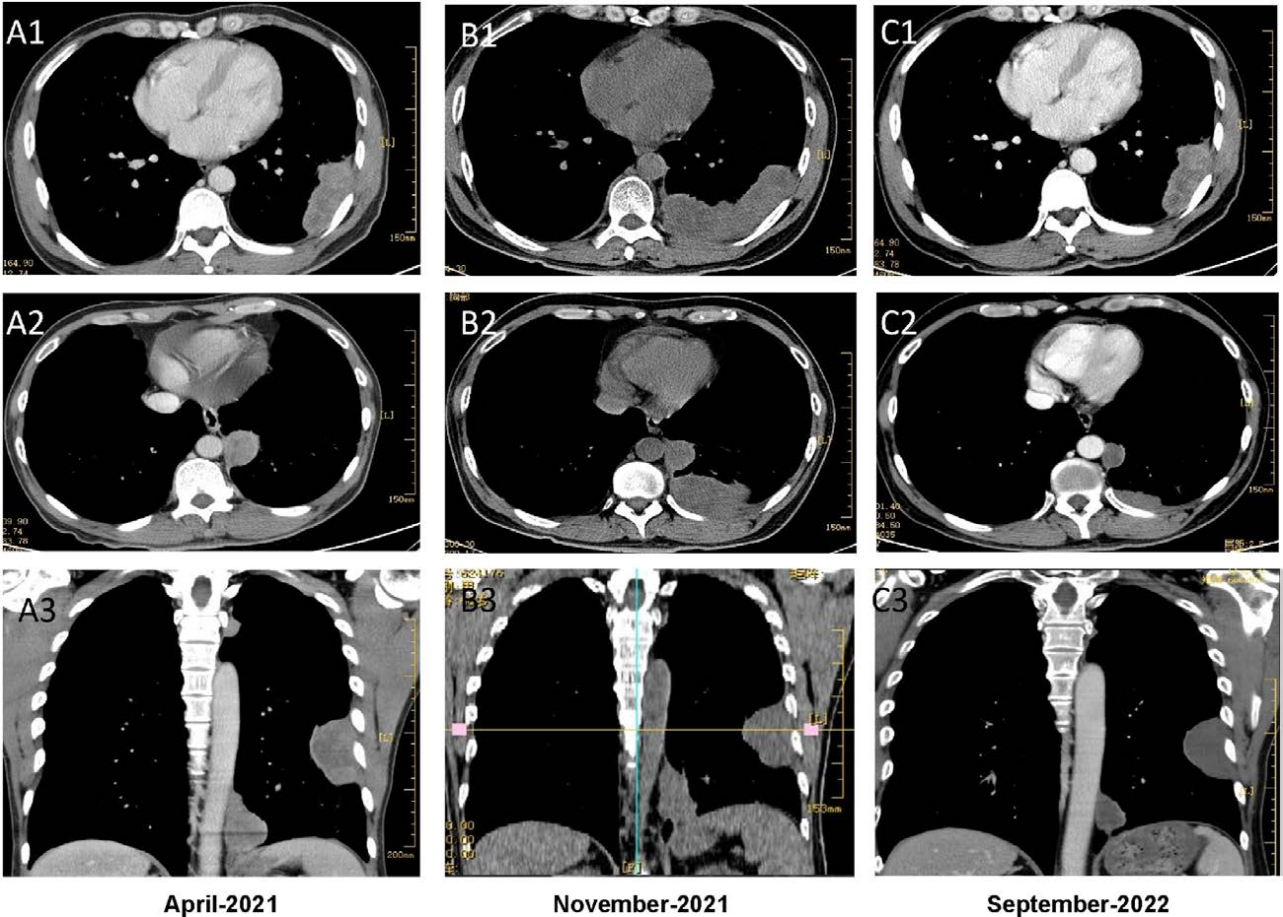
CT changes around treatment. A1–3, before treatment. B1–3, progression after first-line treatment. C1–3, after 10 months of treatment with second-line tirelizumab combined with arrotinib, the lesions were shrunk with reduced solid components.
